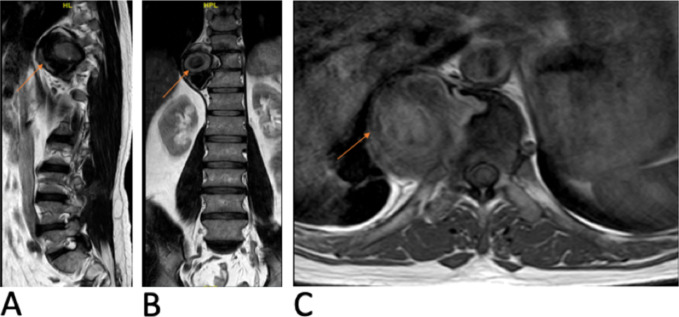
Radiograph of the lumbar and thoracic spine MRI without contrast. A and B, Sagittal and coronal T-2 weighted image showing a right paraspinal lesion with heterogeneous signal and concentric layering. C, Axial T1-weighted imaging showing pulsation artifact.
